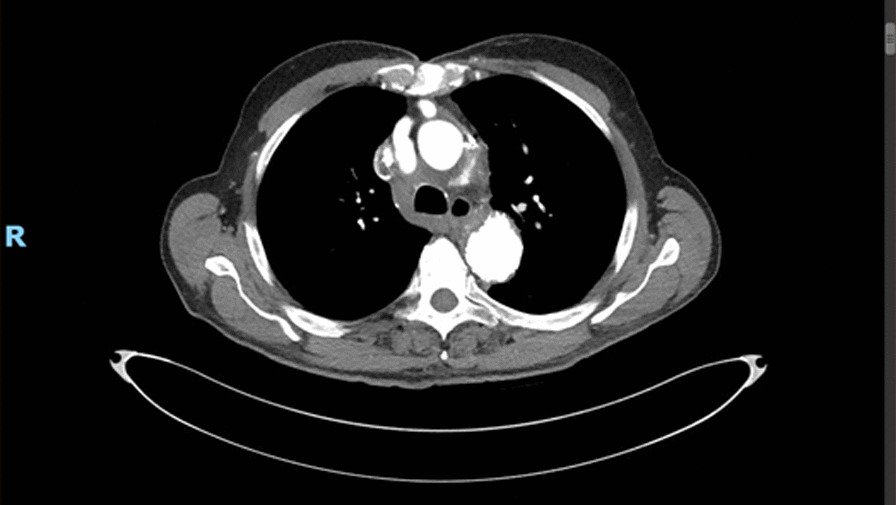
Computed tomography angiogram at 18 months’ follow-up, essentially demonstrating no residual perigraft collection
